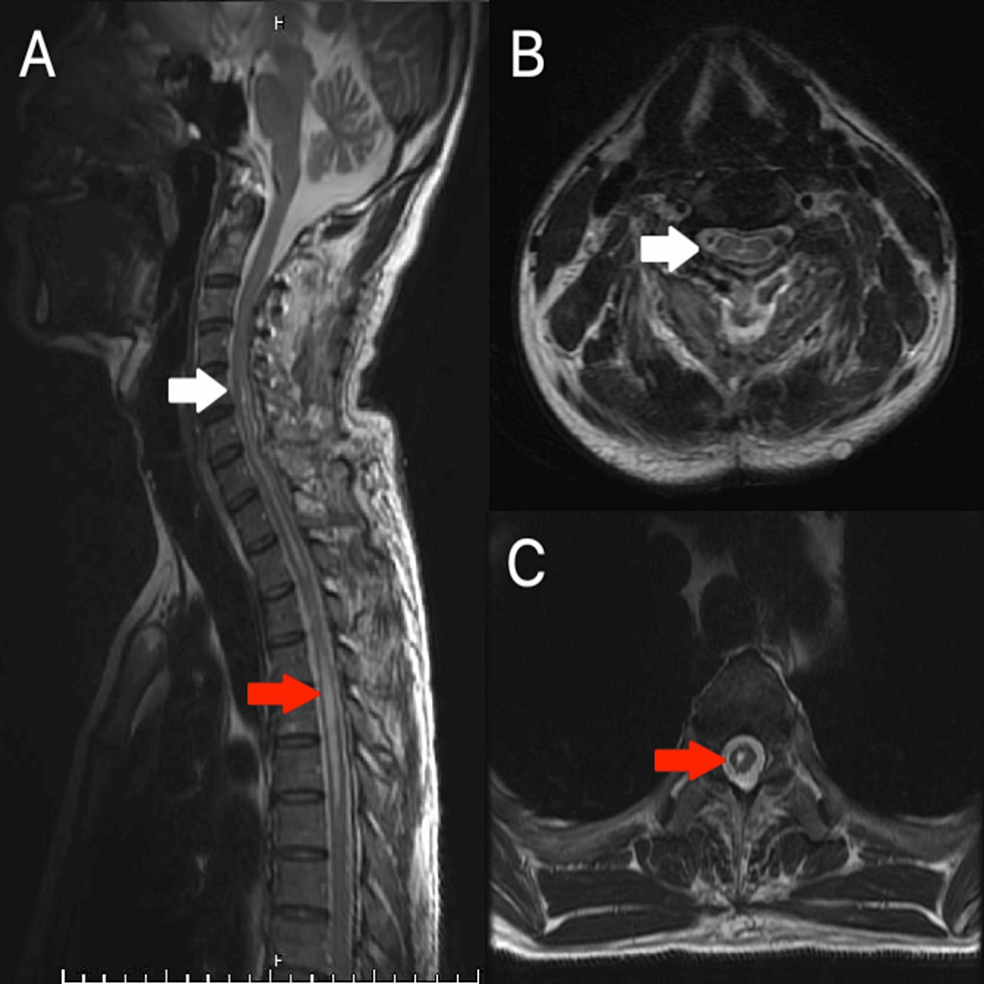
Postoperative T2-weighted MRI. The tumor is completely removed on the sagittal plane (A) and the axial plane at C5 (B, white arrow) and at the T4-T5 level corresponds to the syringomyelia (C, red arrow).
